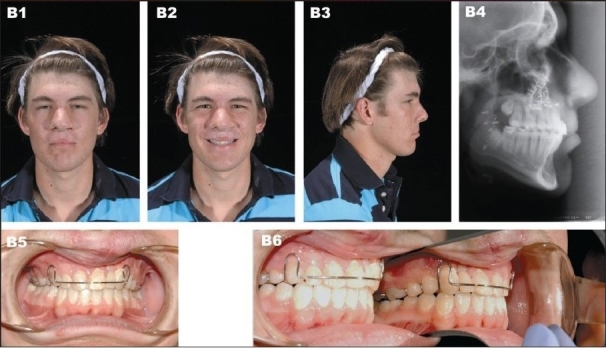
(B) Patient at 20 years of age demonstrating long-term outcome after a four-piece Lefort I osteotomy with maxillary advancement plus bilateral mandibular ascending ramus sagittal split osteotomy. (B1) Frontal view (B2) Frontal smiling (B3) Right lateral view (B4) Postperative lateral cephalometric roentgenogram (B5, B6) Occlusion after completion of treatment
